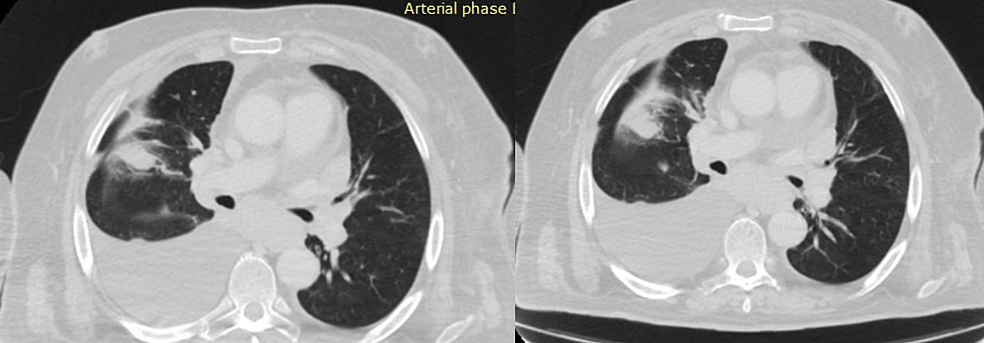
Chest CT of the patient with lymphoma (pleural effusion, pneumofibrosis, consolidation, central lymphadenopathy are presented)
